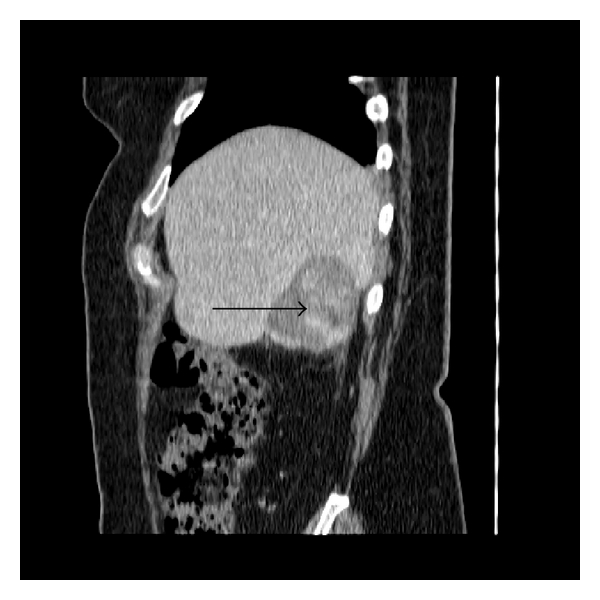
Contrast-enhanced computed tomography of the abdomen in the coronal plane demonstrates intralesional areas of arterial enhancement (arrow). There is a small amount of paracolic gutter fluid.
